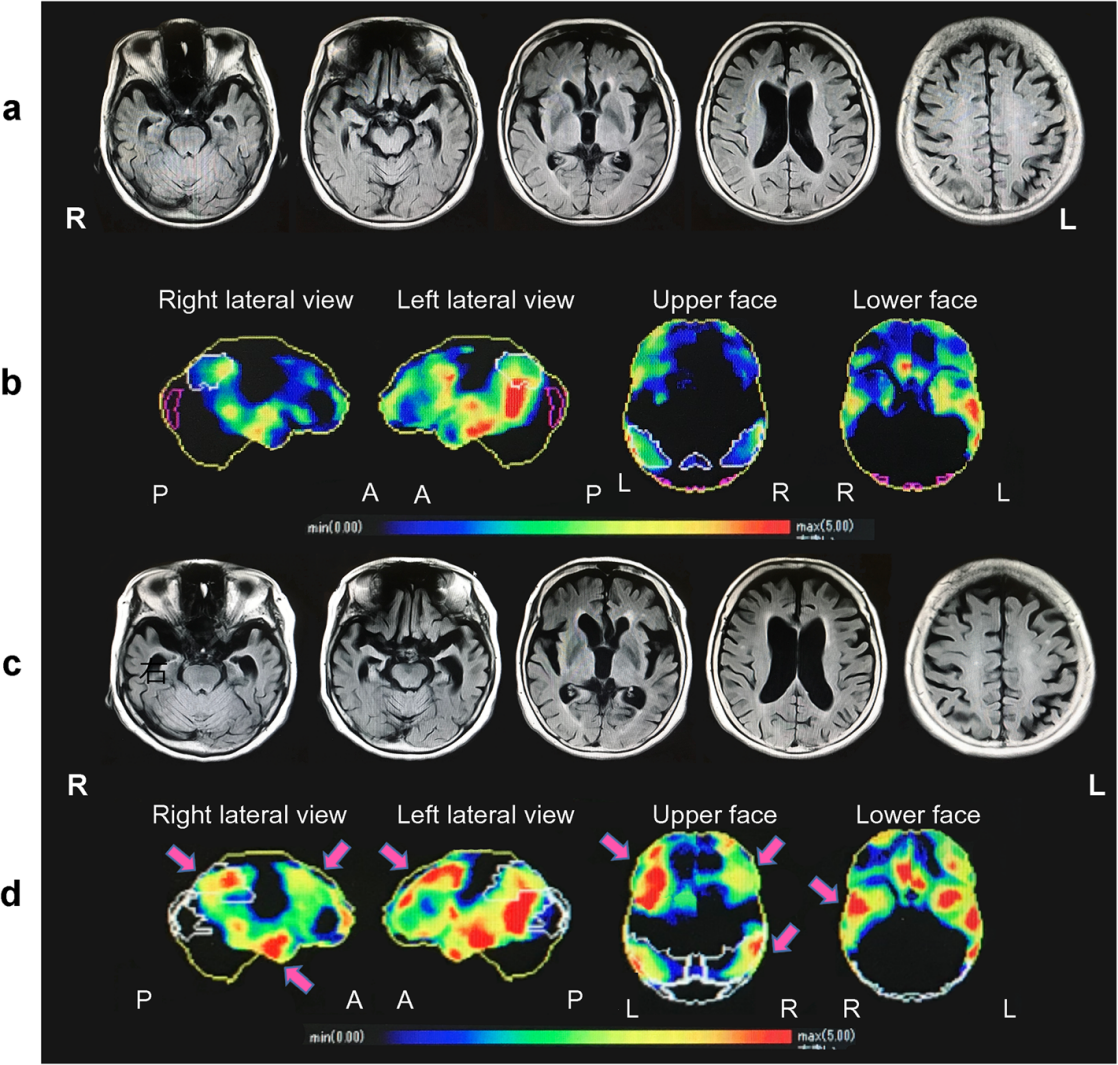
Brain MRI and cerebral blood flow findings at 75 and 77 years of age. At age 75, magnetic resonance imaging (MRI) showed cerebral atrophy of the temporal lobe, parietal lobe, and frontal lobe with dominance in the left cerebral hemisphere, and bilateral ventricular dilatation was also observed (a). In this image, the region and degree of decreased blood flow was objectively evaluated by displaying how many standard deviations the pixel value of the analyzed case deviates from the normal mean value using Z-score [Z-score = (normal mean value − case pixel value)/normal standard deviation]. The Z-score is indicated by 0 (blue) to 5 (red); the higher the value, the stronger the decrease in blood flow. Single-photon emission computed tomography (SPECT) with *N*-isopropyl-p-(iodine-123)-iodoamphetamine (IMP) revealed a reduction of cerebral blood flow in the left hemisphere with slight predominance in the frontal, temporal, and parietal lobes and posterior cingulate gyrus to cuneus (b). At age 77, the brain atrophy extended to the temporal-parietal lobe of the right hemisphere, with bilateral frontal lobe atrophy (c). Consistently, blood flow reduction was spread over a wide area in the right hemisphere, and a reduction in blood flow is observed in the bilateral frontal lobe, temporal lobe, and parietal lobe. Arrows demonstrate the areas of the region where hypoperfusion has expanded (d)
